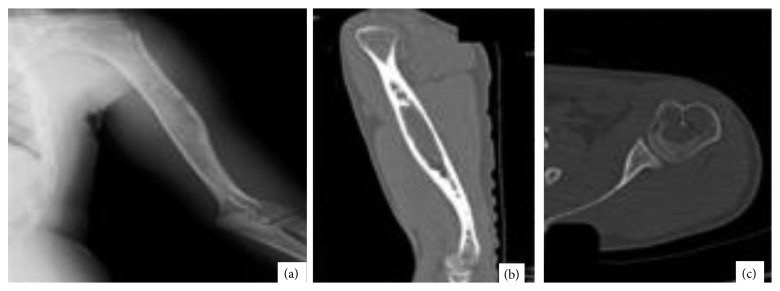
(a) AP X-ray of the humerus showing cystic lesions involving the proximal and midhumerus. (b, c) Sagittal and axial CT for the same patient showing expansile cysts of the mid and proximal humerus, respectively.
